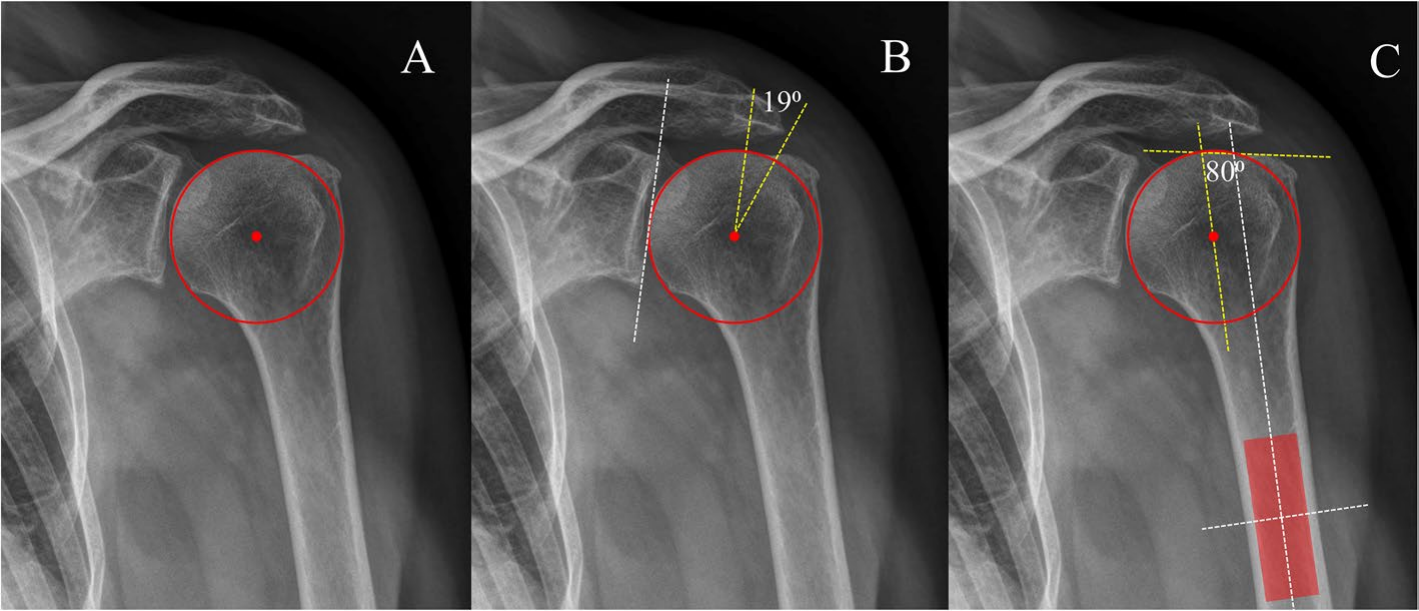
On a true AP glenohumeral X-ray, A the humeral head is circled, and the center of rotation is marked. B Measuring the ACEA formed with the first drawn superiorly from the center of the humerus parallel to the glenoid surface and the second drawn from the center of the circle to the lateral-most aspect of the acromion edge. C Measuring the GTA angle formed by the first drawn parallel to the diaphyseal axis that passes through the humeral head center of rotation; the second drawn connects the superior border of the humeral head to the superolateral edge of the greater tuberosity
